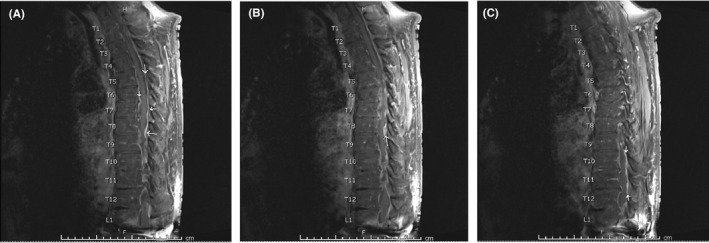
(A–C). MRI with contrast displaying abnormal meningeal enhancement in the mid‐ to lower thoracic spine
